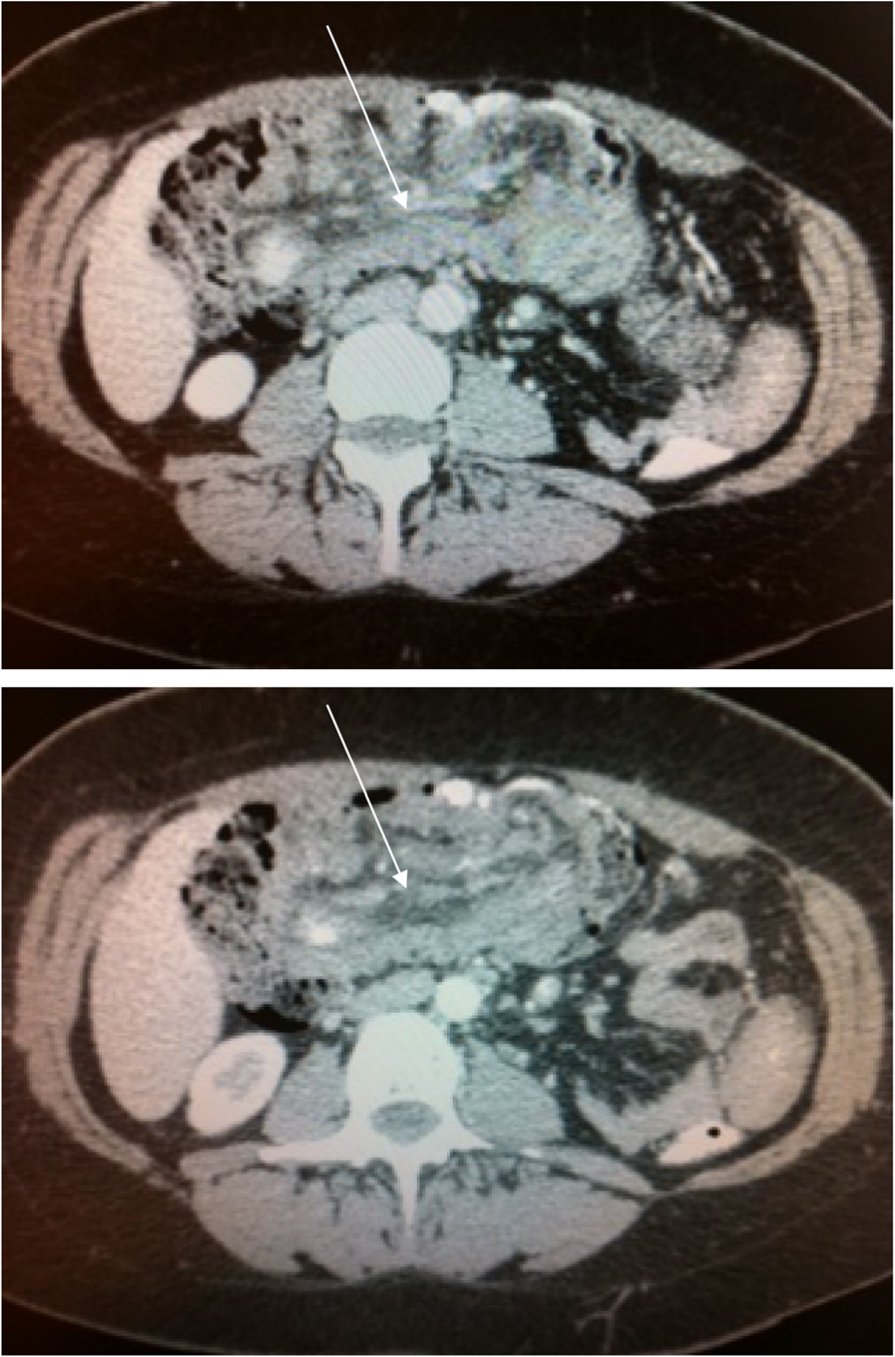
The computed tomography scan shows one an ileocolocolic intussusception.
